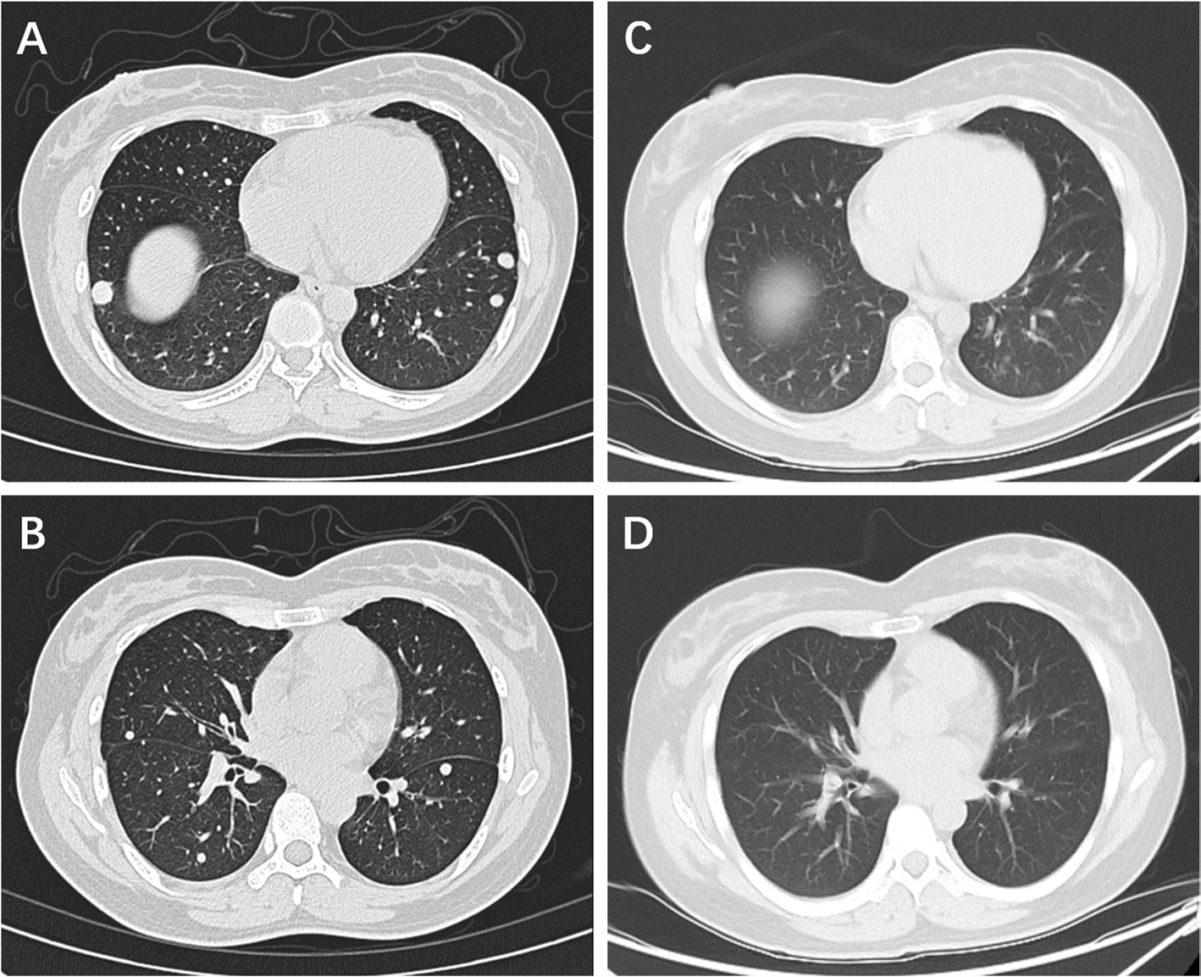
Radiological changes for the patient (PR) with metastatic alveolar soft part sarcoma. A, B Before treatment computerized tomography (CT) scans of target lesions in the lung site. C, D After treatment CT scans of target lesions in the lung site
